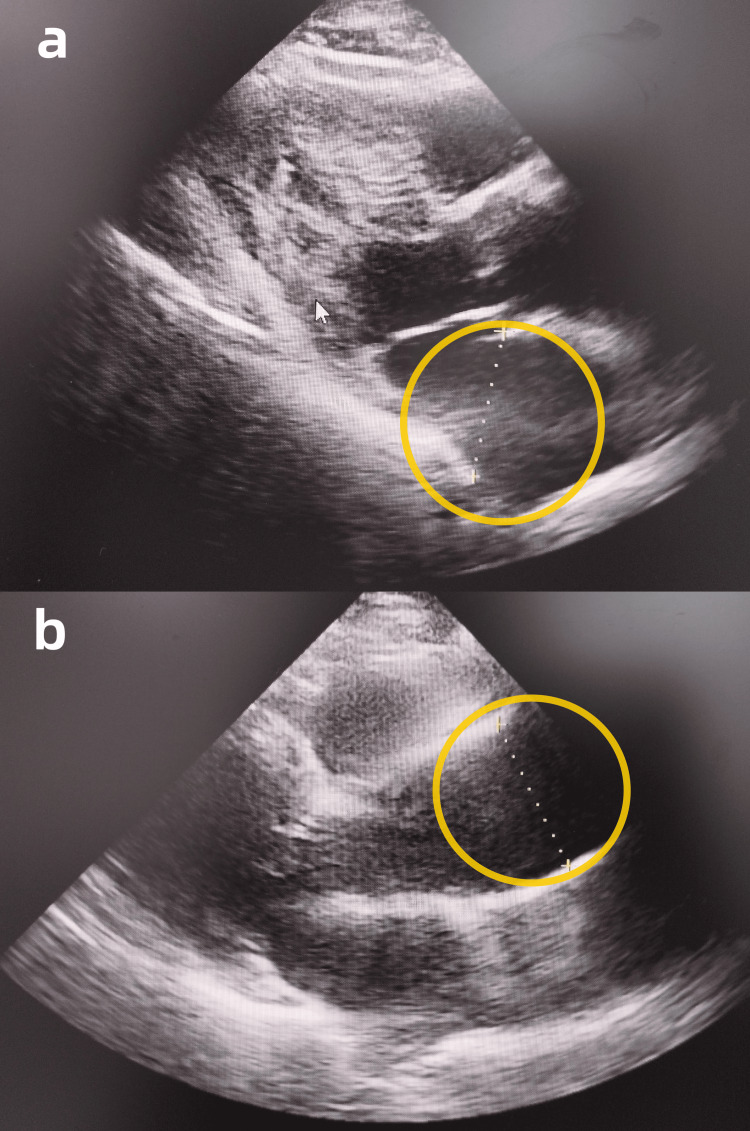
Echocardiography (a) The yellow circle indicates mild left atrial enlargement. (b) The yellow circle indicates a dilated ascending aorta.
